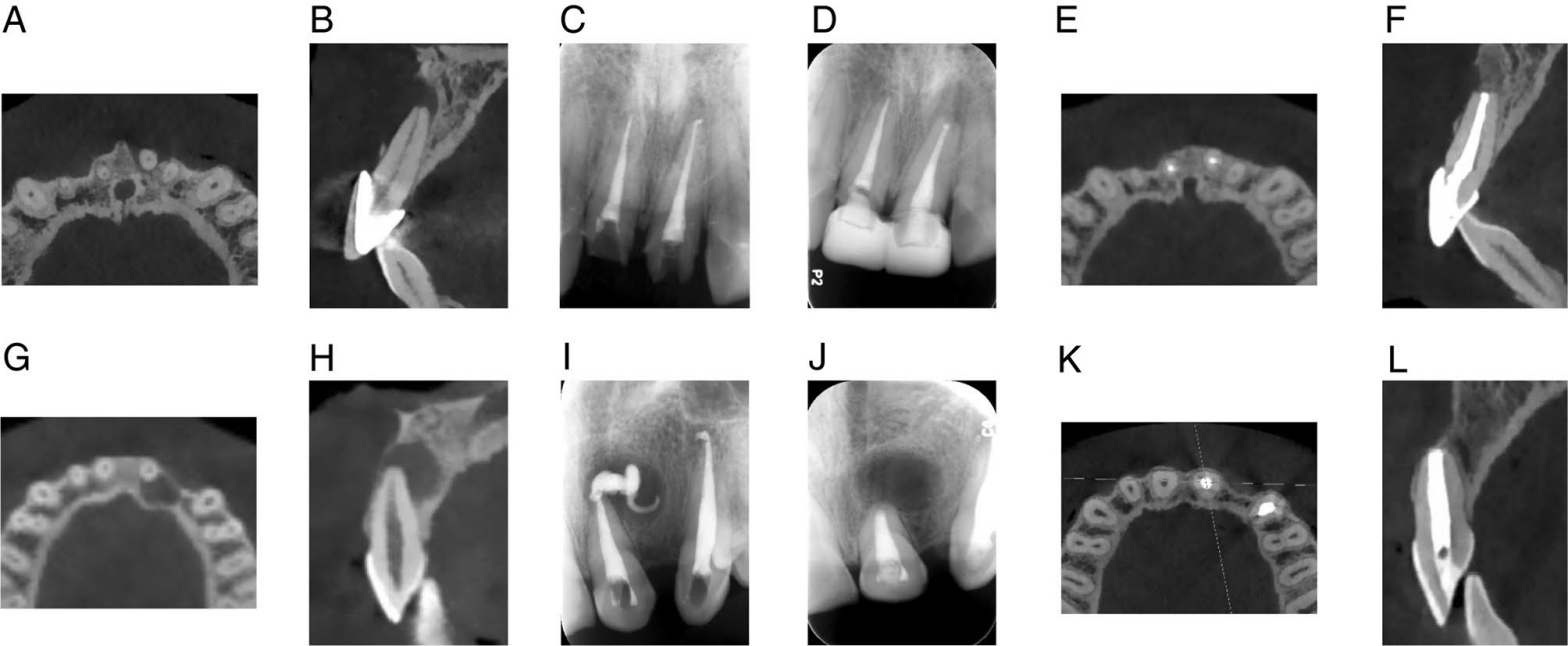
Two cases of endodontic microsurgery with 3D printed surgical guide. Case 1: Preoperative radiographic imaging (A,B), periapical radiograph after root canal treatment (C), periapical radiograph right after microsurgery (D) and radiographic imaging recalled after 12 months (E,F). Case 2: Preoperative radiographic imaging (G,H), periapical radiograph after root canal treatment (I), periapical radiograph right after microsurgery (J) and radiographic imaging recalled after 12 months (K,L).
